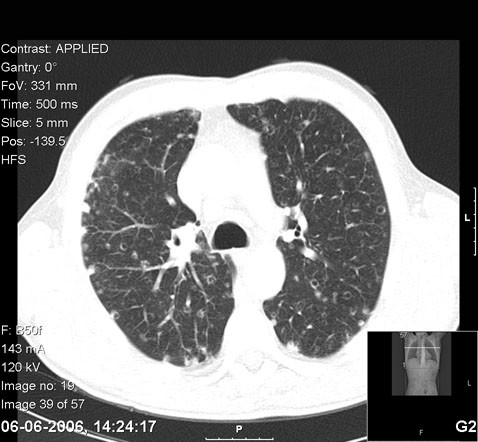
Thoracoabdominal-pelvic computed tomography scan of the patient, showing multiple cysts and nodules and interstitial thickening in the lungs.
